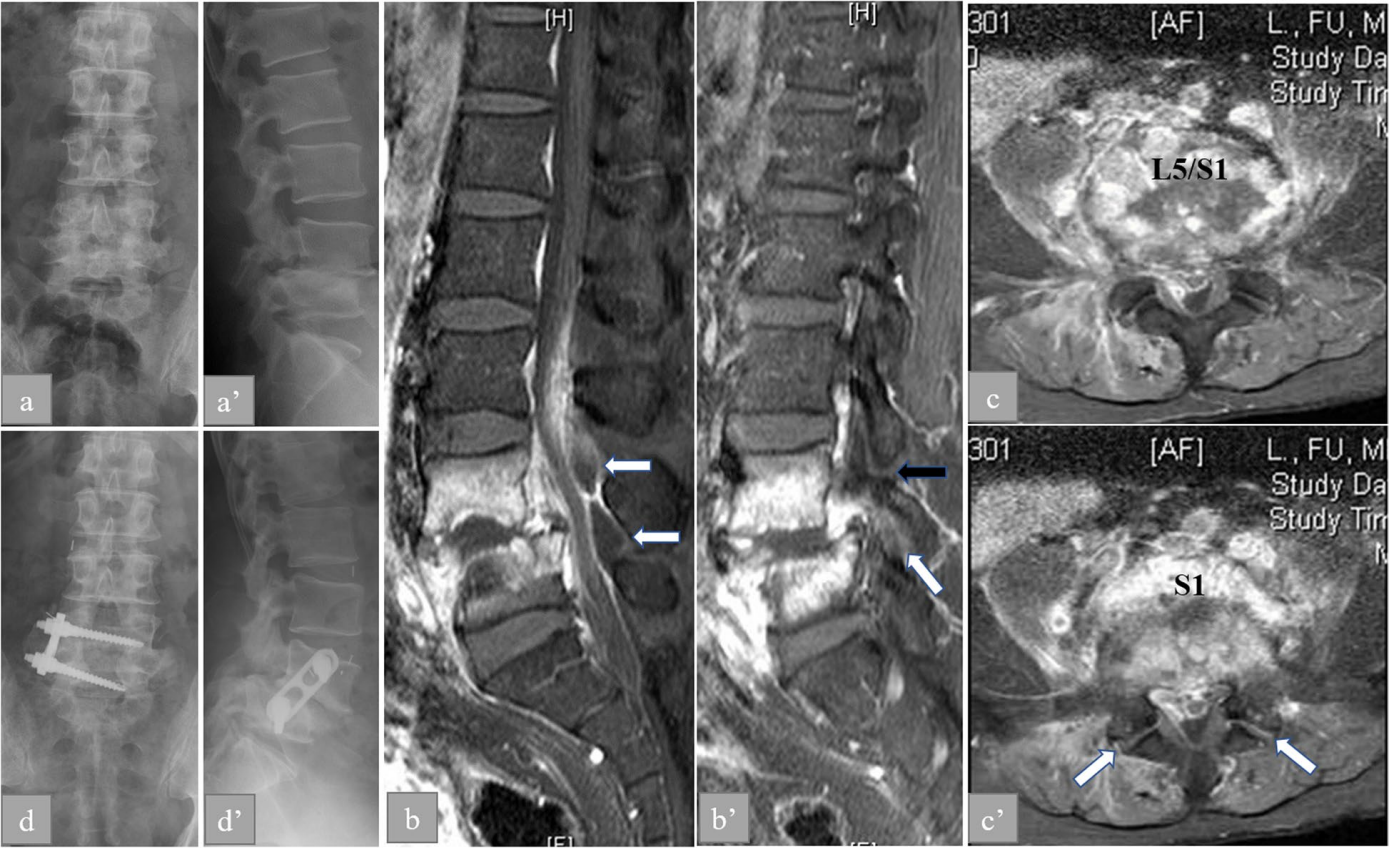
Anaerobic spondylodiscitis with concomitant spondylolytic spondylolisthesis in a 53-year-old male (No. 1). A1, 2 Grade 2 spondylolisthesis with pars interarticularis deficiency at L5–S1 observed on plain radiographs. B1 Infectious spondylodiscitis at L5–S1 with a destroyed disk on sagittal enhanced T1-weighted magnetic resonance imaging (MRI). The dorsal epidural abscesses are visible (white arrows). B2 Heterogeneous enhancement of the lumbosacral facet joint indicating that the facet joint was destroyed through infection (white arrow). Collection of purulent pus extended to the pars interarticularis deficiency (black arrow). C1, 2 Heterogenous enhancement of L5–S1, prevertebral space, bilateral foramina, and spinal canal. The collection of pus was acuminated in the lumbosacral facet joint space (white arrow). D1, 2 Anaerobic spondylodiscitis with concomitant spondylolytic spondylolisthesis diagnosed through contrast-enhanced MRI and bacterial cultures. The patient underwent anterior sequestration and reconstruction with interbody fusion with autogenous iliac crest and instrumentation. The solid bony fusion without the loosening of the instrumentation was observed at the 2-year follow-up
